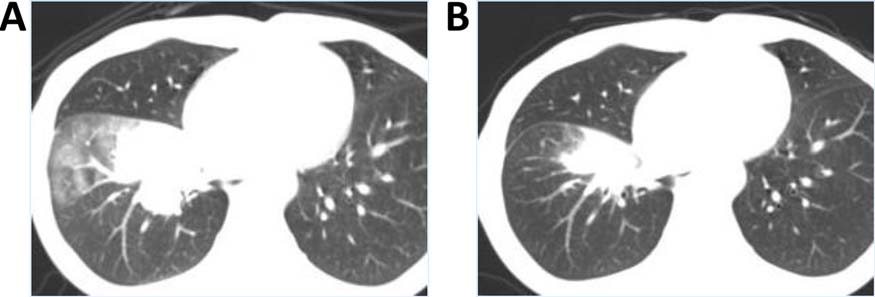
(A) CT before treatment. Obstructive pneumonia was found in the right lower lobe. Transarterial chemo-embolization through two right bronchial arteries and right inferior phrenic artery was performed using 30 mg of cisplatin and 500 mg of 5-FU with 5 mg of HepaSphere (50–100). (B) CT in 2 months after treatment. Obstructive pneumonia was markedly improved according to shrinkage of tumor invading the pulmonary hilum. Symptoms caused by obstructive pneumonia were improved.
